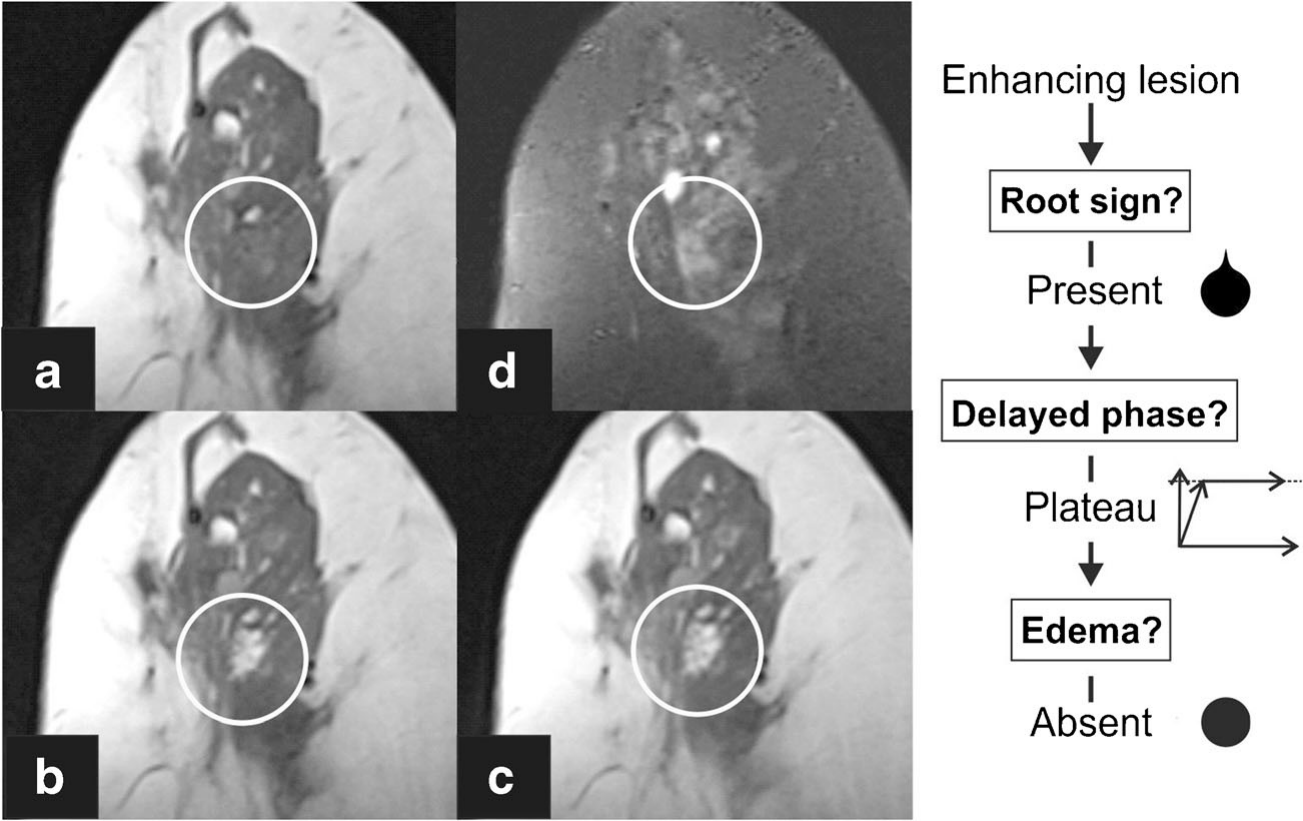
Apocrine ductal carcinoma in situ (DCIS) Grade 2 presenting as a mass lesion with the root sign, delayed plateau enhancement and perifocal oedema, resulting in a classification as *Tree* node 10. Representative axial slices of the T1-weighted, non-enhanced sequence (a), a T2 TIRM sequence (b), early (c) and delayed (d) post-contrast T1-weighted sequences are shown
